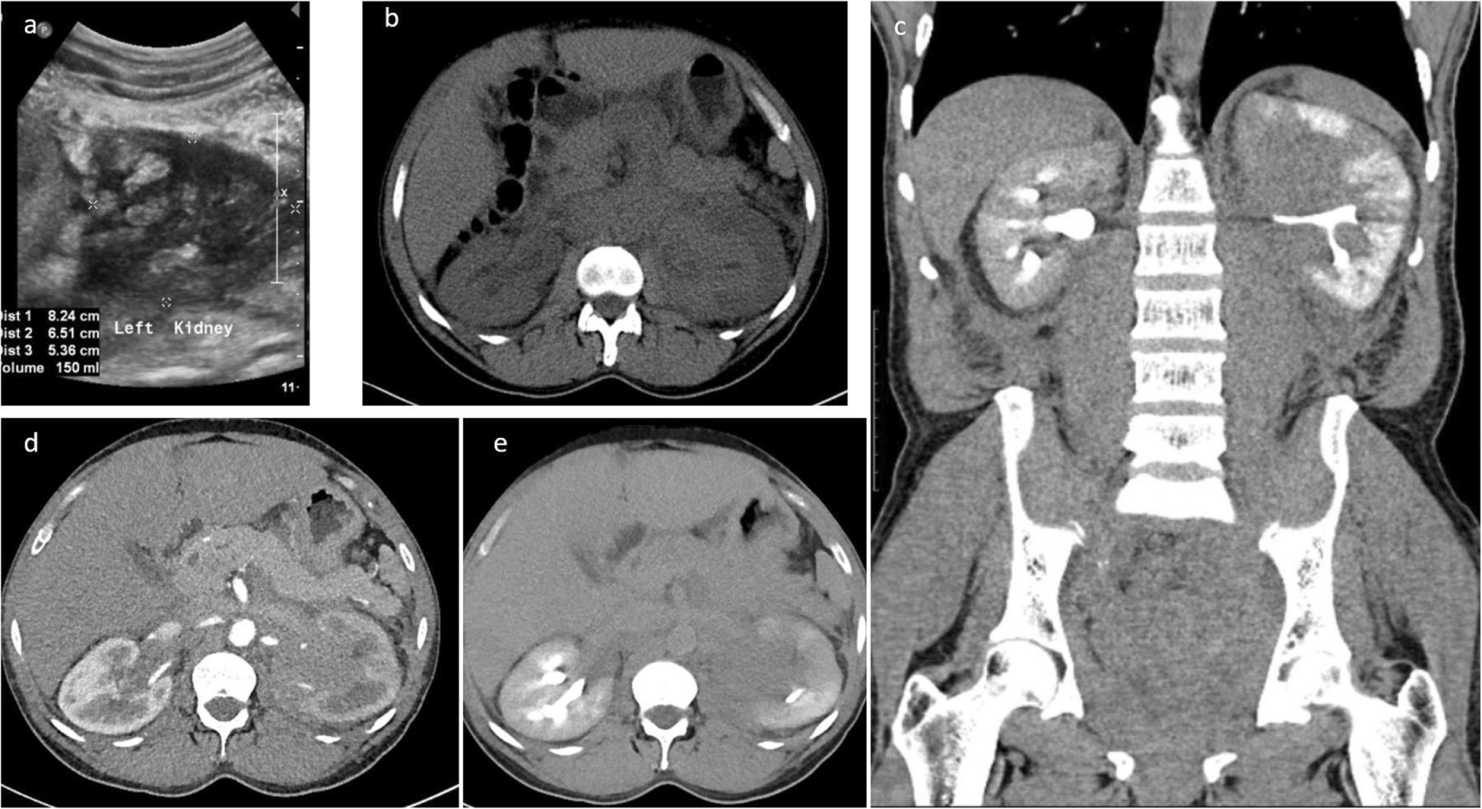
Ultrasound image of the left kidney in a HIV-positive male demonstrates heterogeneously echogenic areas in the medulla extending to the collecting system. Unenhanced CT KUB showed diffuse thickening of the renal pelvis with hyperdense soft tissue material relative to the renal parenchyma. Heterogenous infiltration of the kidney is seen on the postcontrast CT images in arterial and urographic phases
